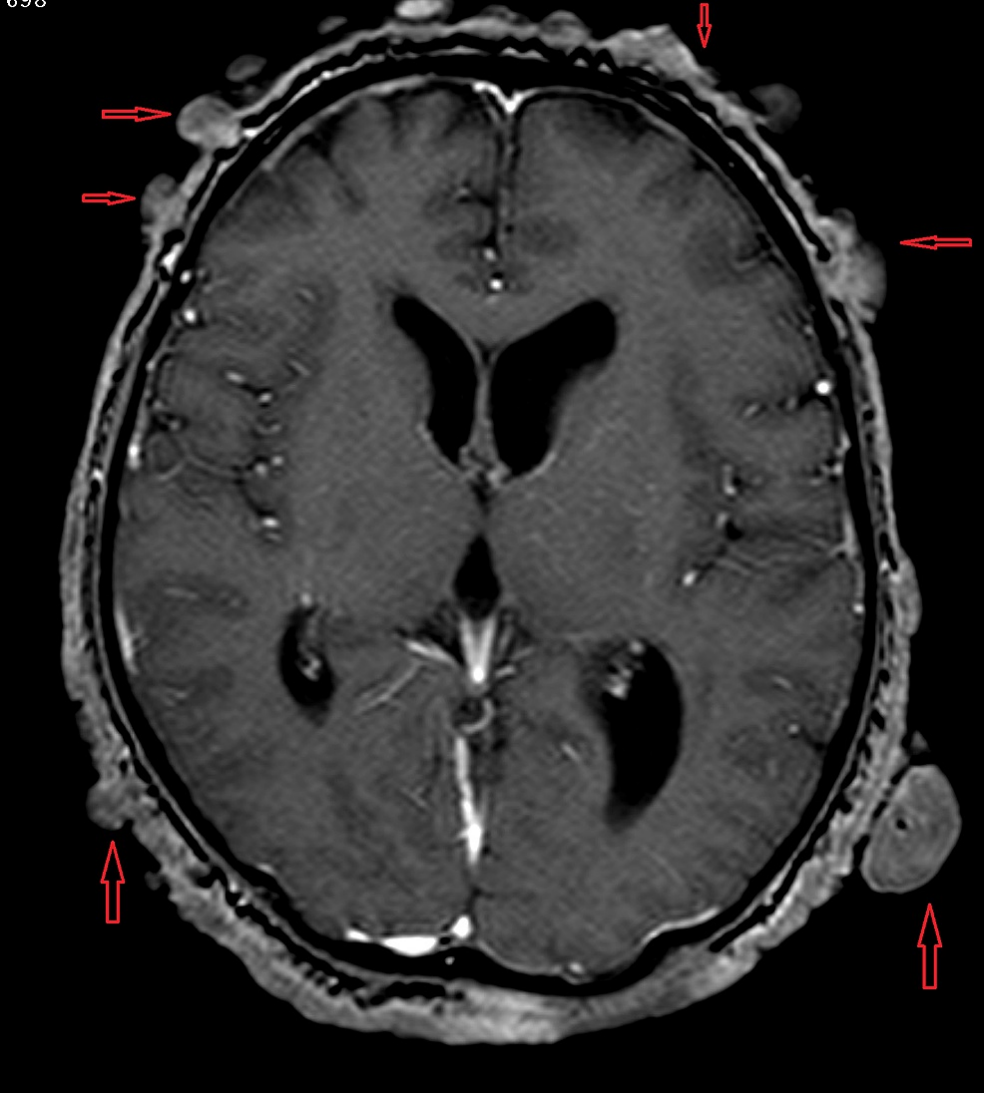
Axial post-contrast computed tomography scan of the head showing multiple growths (red arrows) on the scalp.
